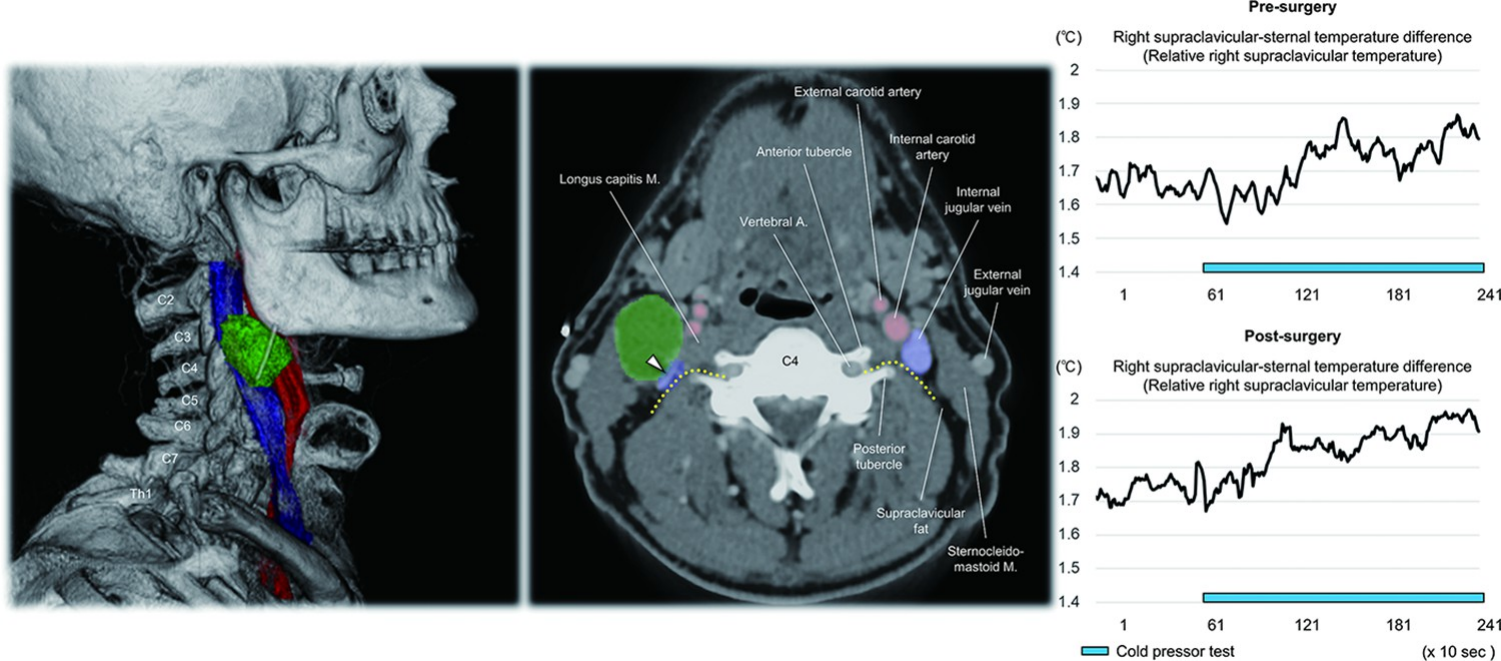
Patient with a cervical tumor and infrared thermography finding. Volume-rendered image of the computed tomography viewed from the right lateral direction (left) shows cervical tumor (green) adjacent to the right internal jugular vein (blue) and carotid artery (red). The location of the tumor is 3^rd^ (C3) to 4^th^ (C4) cervical nerve level. Horizontal section viewed from the inferior direction (middle) confirms compression of the right internal jugular vein (white arrowhead), which is also close to the estimated location of the proximal segment of the C4 (yellow dotted lines) just lateral to the longus capitis muscle. Right panels show the pre- and post-surgical result of the infrared thermography of the right supraclavicular region. The increase in the relative temperature (difference between the sternal and supraclavicular temperatures) in response to the cold pressor (blue bar) increased further after surgery (Δ0.164°C to Δ0.270°C). A, artery; M, muscle.
